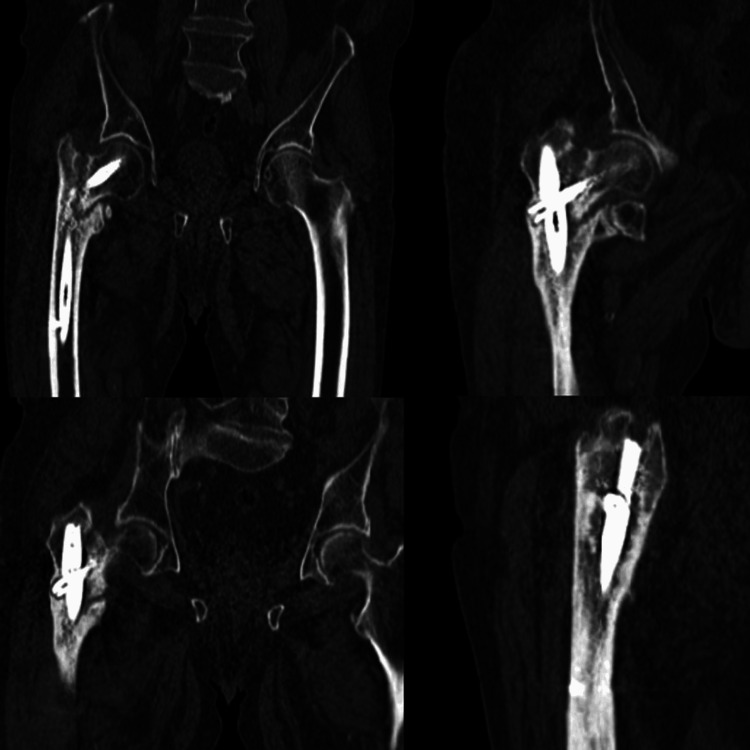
Computed tomography scan demonstrating nonunion of a pertrochanteric fracture with fatigue failure and breakage of the Gamma cephalomedullary nail
